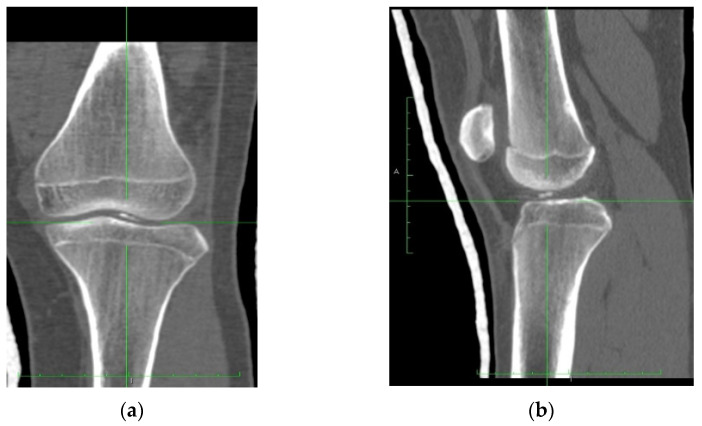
CT scans of the injured knee clearly showing the fractured fragment; (a) AP view and (b) L view.
